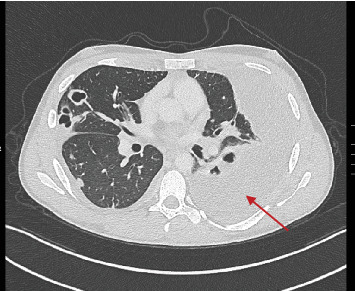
CT scan showing pulmonary excavated nodules with left pleural effusion.
